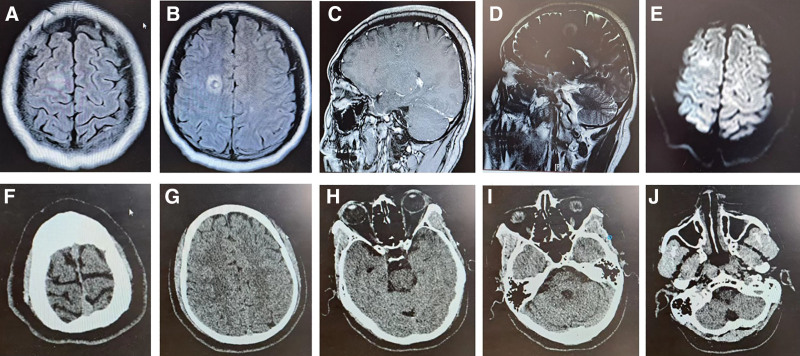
Changes in imaging of head bleeding lesions in patients before and after treatment. (A–E) Changes in MRI at patient admission. (H–J) Head CT changes during follow-up 6 mo after discharge. CT = computed tomography, MRI = magnetic resonance imaging.
